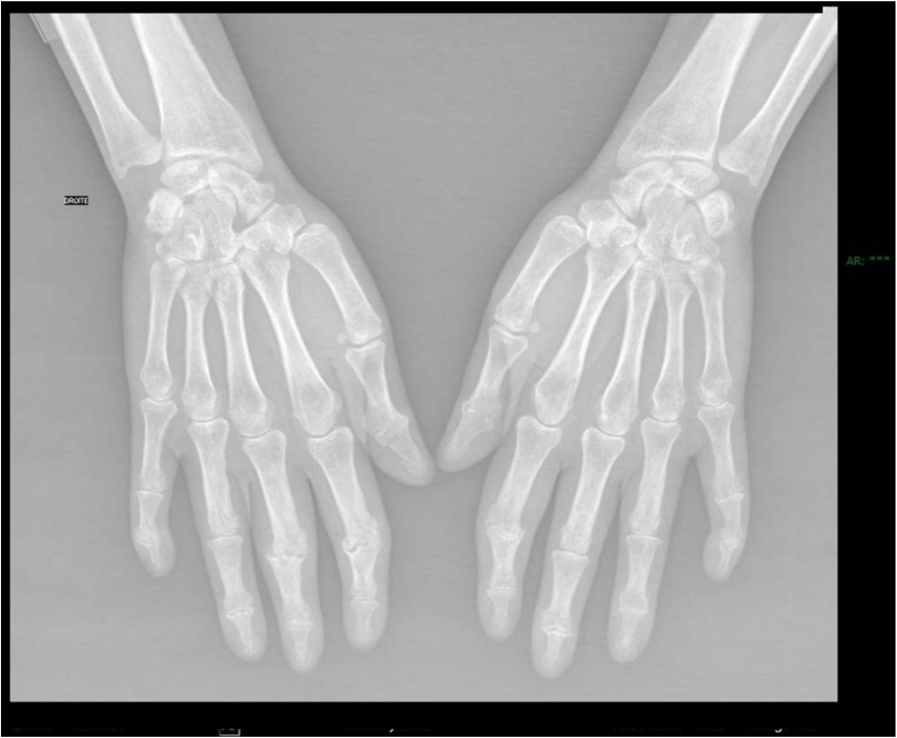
Magnetic resonance imaging scanner of the right hand showing an advanced and destructive arthropathy associated with significant synovitis of the proximal interphalangeal joints of the first, second, and third rays and, to a lesser extent, of the fourth and fifth rays
